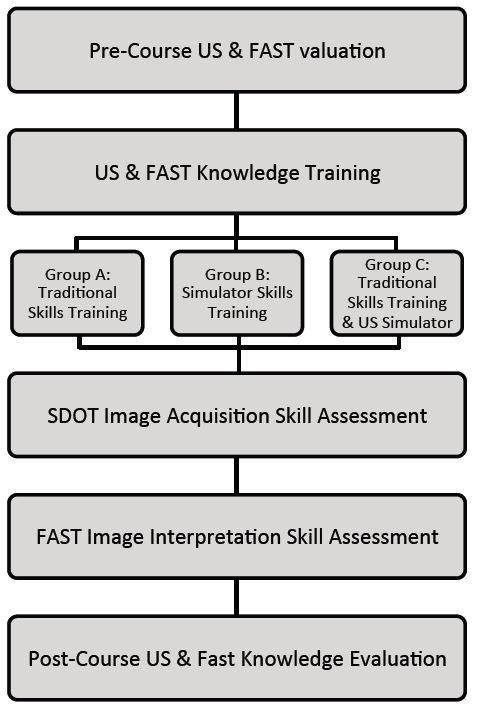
Study design. *US,* ultrasound; *FAST*, focused assessment of sonography in trauma; *SDOT*, standardized direct observation
